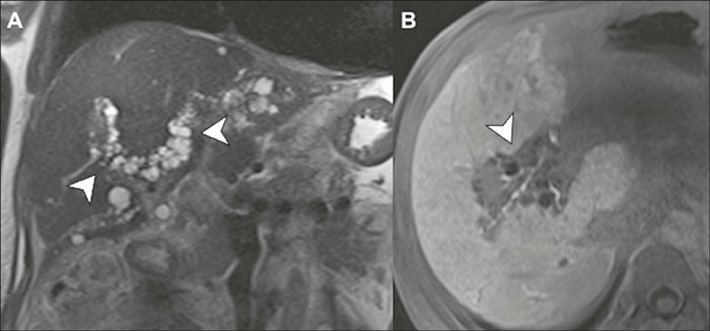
Peribiliary cysts. Coronal T2-weighted MRI scan (A)
